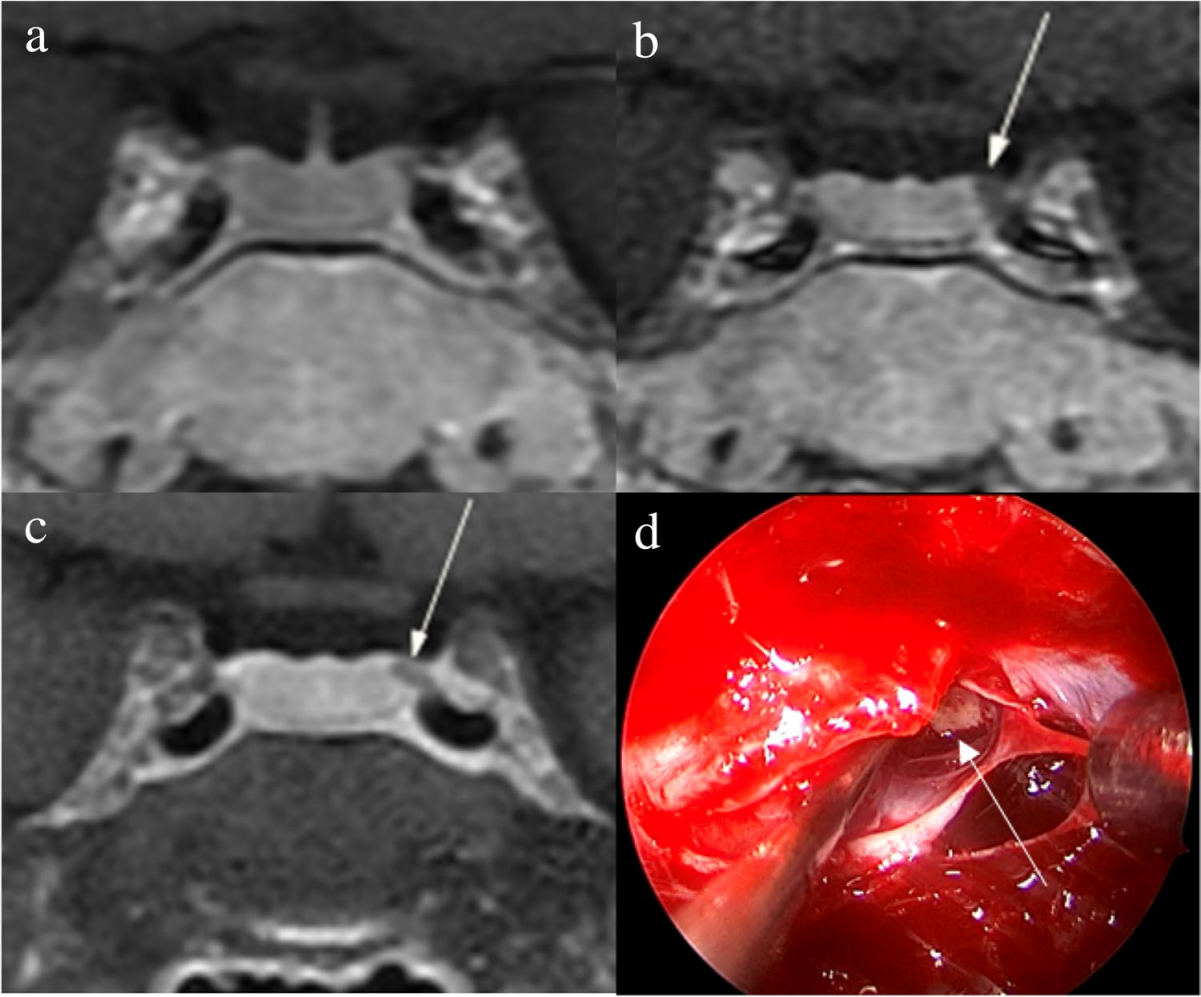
Images in a 34-year-old woman with Cushing’s disease. No tumor is identified on (a) coronal contrast-enhanced T1-weighted image obtained with two-dimensional (2D) fast spin echo (FSE) sequence. The 3-mm pituitary microadenoma (arrow) with delayed enhancement is identified on the left side of the pituitary gland on (b) coronal dynamic contrast-enhanced T1-weighted image obtained with 2D FSE sequence and (c) coronal contrast-enhanced T1-weighted image on high-resolution MRI obtained with 3D FSE sequence. d Intraoperative endoscopic photograph during transsphenoidal surgery shows a 3-mm pituitary microadenoma (arrow)
